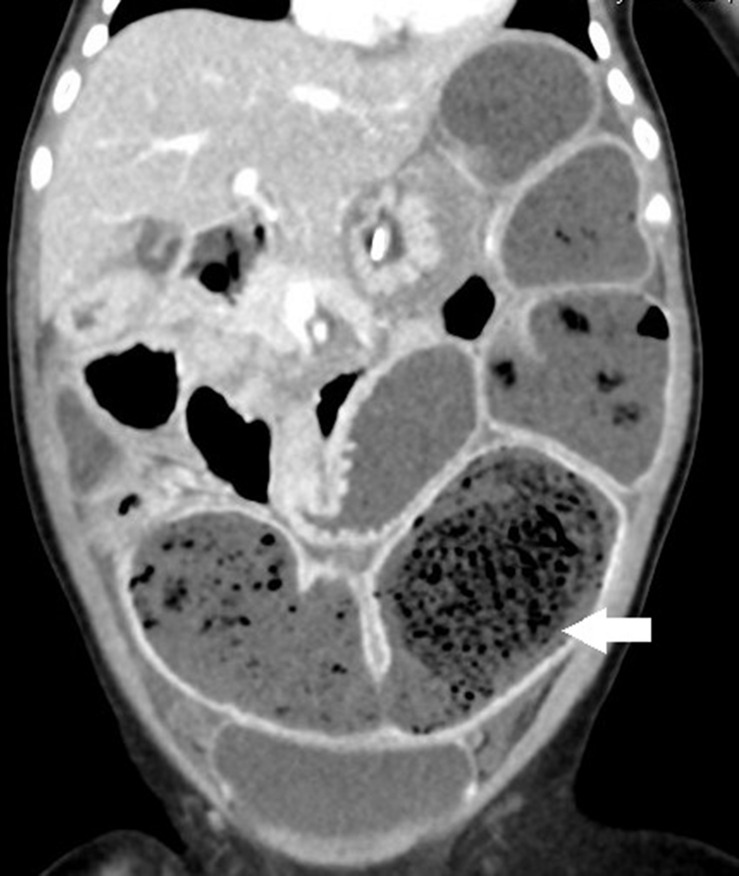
The coronal section of abdominal computed tomography (CT) discloses a prominent dilated bowel loop with air bubble-interspersed feces (lactobezoar) at the left lower quadrant (white arrow).
